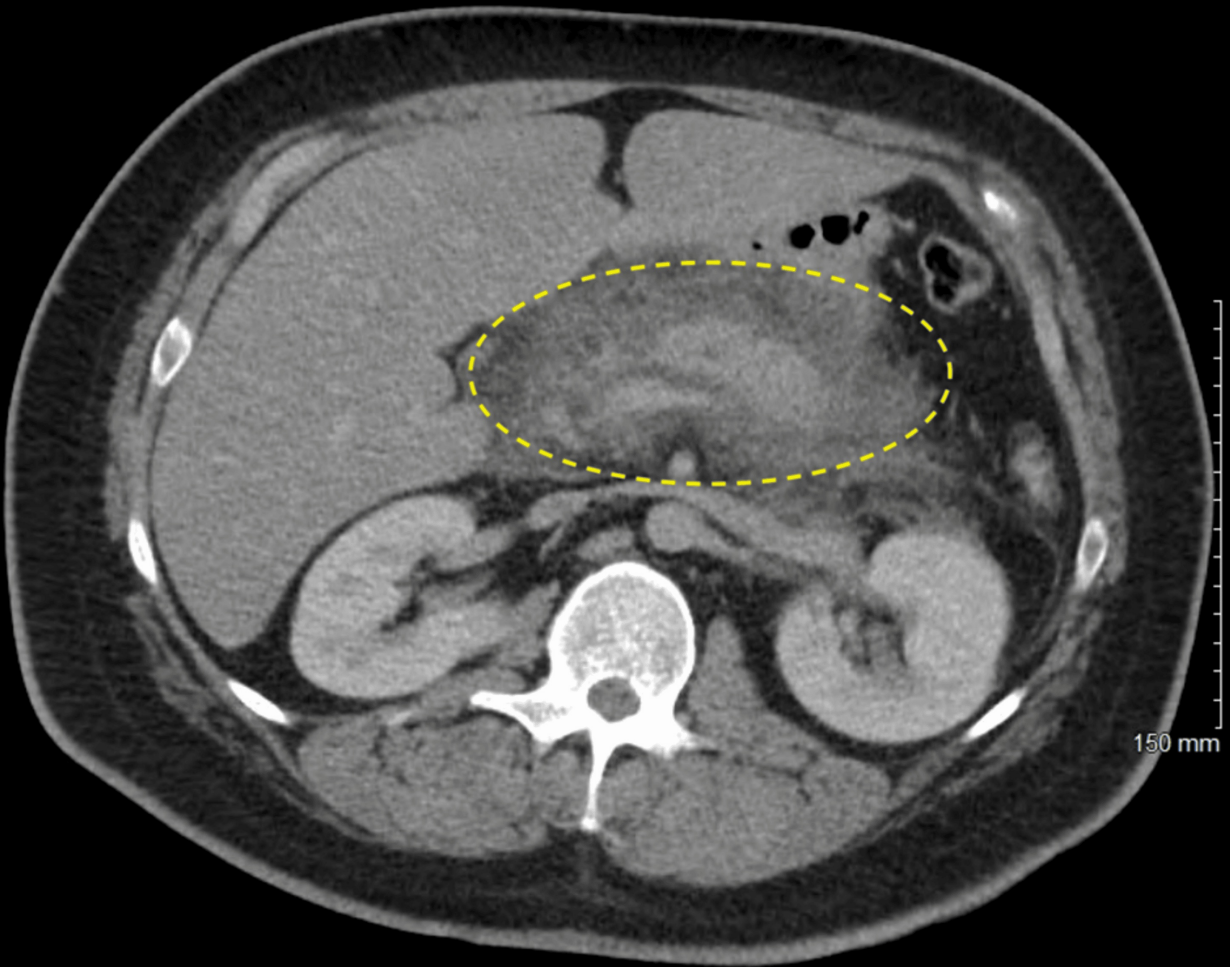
Axial section of computed tomography reveals pancreatitis and peri-pancreatic inflammation
